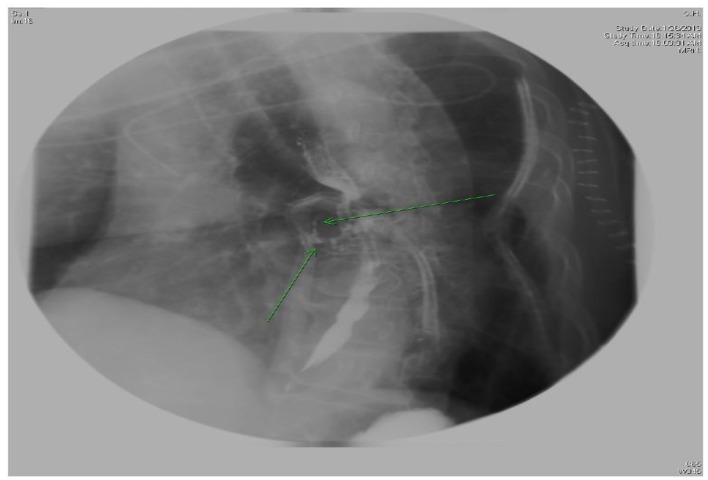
X-ray esophagus with gastrografin showing two sinus tracts extending from anterior aspect of esophagus at level T7.
